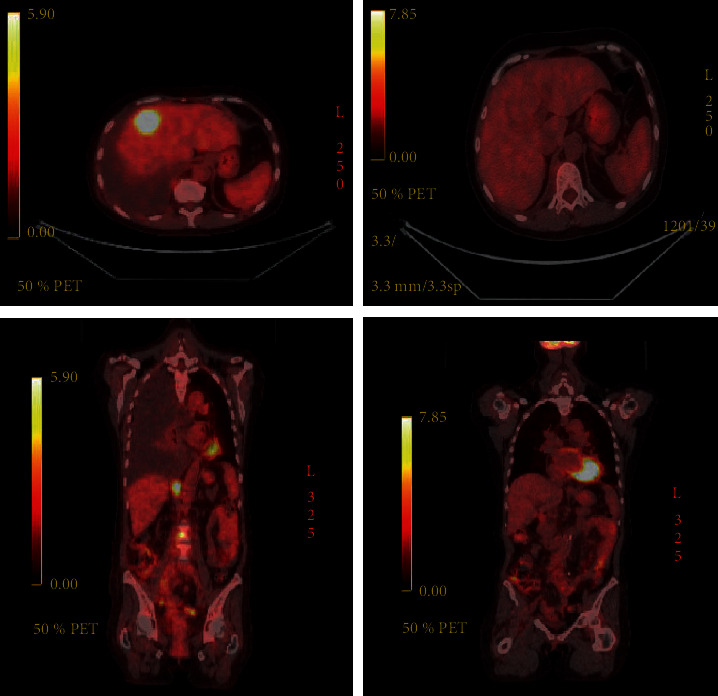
PET/CT scans showing improvement in metastatic disease with reduction in hepatic mass and decreased retroperitoneal, pelvic, and iliac lymphadenopathy after 4 cycles of pembrolizumab.
